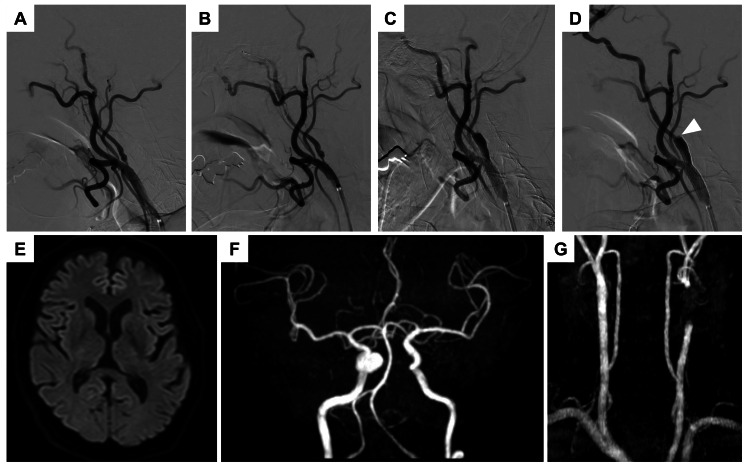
Intraoperative angiograms and postoperative imaging findings of CAS A: Preoperative left CCA angiogram; B: Left CCA angiogram showing the protection filter placed in the left ICA; C: Left CCA angiogram immediately after placement of an open-cell stent; D: Final angiogram of CAS. The arrowhead indicates contrast stagnation; E: DWI performed on the day after CAS, showing no high-intensity lesions in the left cerebral hemisphere; F-G: Head and neck MRA performed the day after CAS, demonstrating normalization of the ICA visualization. CAS: carotid artery stenting; CCA: common carotid artery; ICA: internal carotid artery; DWI: diffusion-weighted imaging; MRA: magnetic resonance angiography
